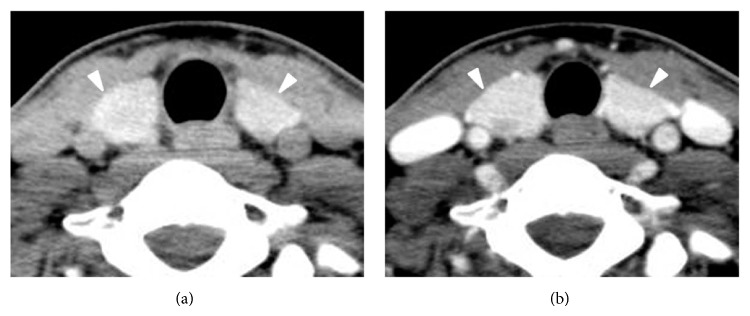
Normal thyroid designated “normal” CT diagnosis in a 28-year-old woman (papillary thyroid carcinoma in the left thyroid). The thyroid gland shows iso- and homogeneous attenuation in nonenhanced CT image (a) and anteroposterior diameter of 1-2 cm, smooth margin, and homogeneous enhancement in contrast-enhanced CT image (b).
